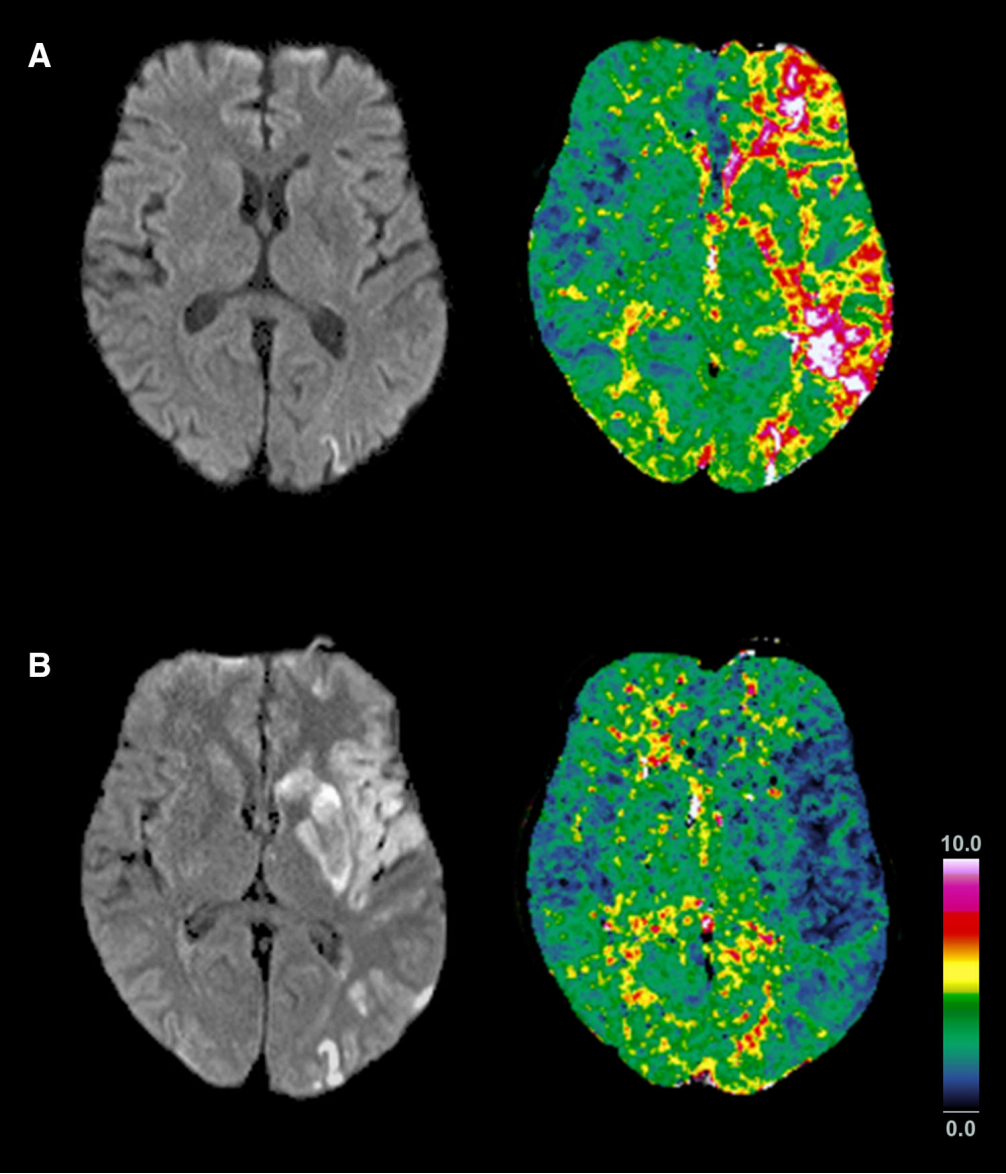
Abnormal yawning without initial DWI restrictions. (a) Evolution of the penumbra in a patient with abnormal yawning initially not related to DWI lesions in the caudate head or insula (Pat N^o^ 4). While cortical DWI restrictions were initially restricted to the frontal lobe (not shown) and parietal lobe, perfusion imaging revealed a widespread penumbra along the left MCA encompassing the insula and caudate head (TTP delay >4.5 s). (b) Follow-up after 48 h revealed prolonged infarction of the tissue at risk in the left insula, striatum and frontal and parietal lobe, now including the caudate head and the insula, with luxury perfusion of the infarcted tissue
